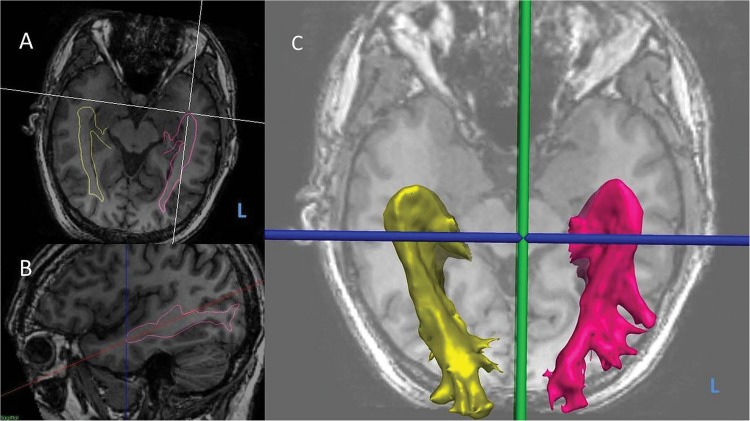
Measurement of optic radiation tractography asymmetry.L, left. (A) Oblique axial view of three-dimensional (3D) T1-weighted MRI with optic radiation tractography surface models (yellow-right, pink-left) overlaid. (B) Sagittal view of right optic radiation surface model (pink) with axial plane tilted to longitudinal axis of hippocampus, and Meyer's loop-temporal pole distance shown (dotted blue line). (C) 3D surface models of left and right optic radiations.
